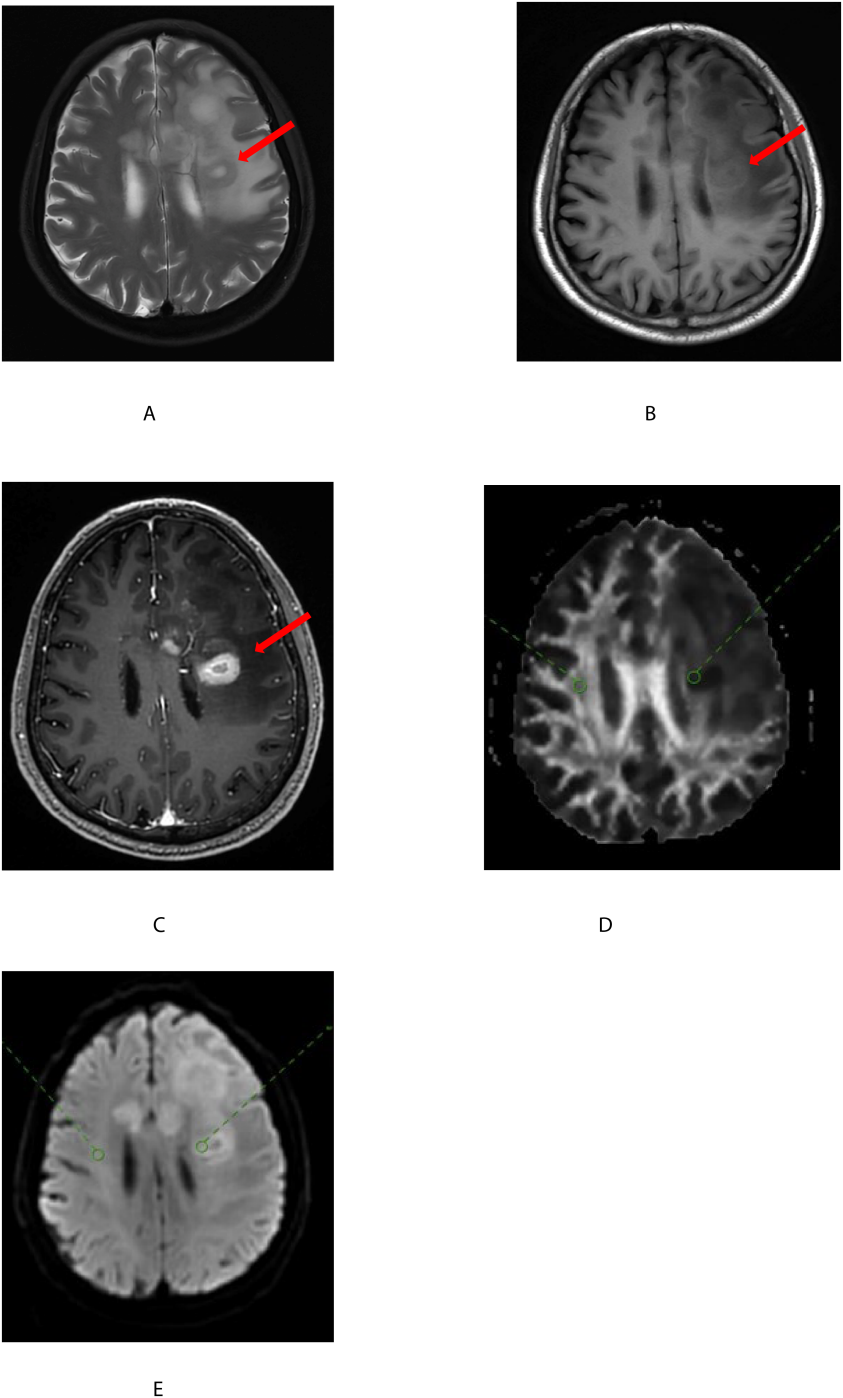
A 68-year-old man with a glioblastoma of the left basal ganglia(shown by the red arrow). T2-weighted images (A) on plain scan, T1-weighted images (B) on plain scan, and T1-weighted images (C) on enhanced scan showed uneven tumor signals with central necrotic foci. On FA (D) and MD (E), two regions of interest (ROI) were placed within the solid portion of the tumor(shown by the red circle) and normal white matter(shown by the green circle) and combined with enhanced T1-weighted images were registered.
